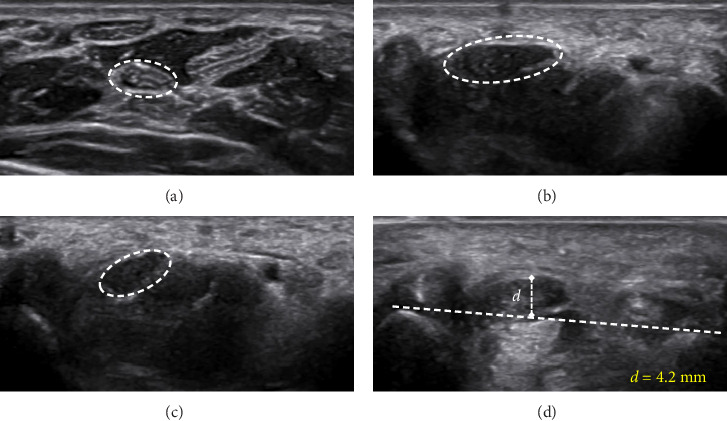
Transverse ultrasound image of the right median nerve in a male patient with severe CTS. (a) CSAp is 0.08 cm^2^. (b) CSA at the carpal tunnel inlet is 0.21 cm^2^. (c) CSA at the outlet is 0.14 cm^2^. (d) Bowing of the flexor retinaculum at the inlet is 4.2 mm.
